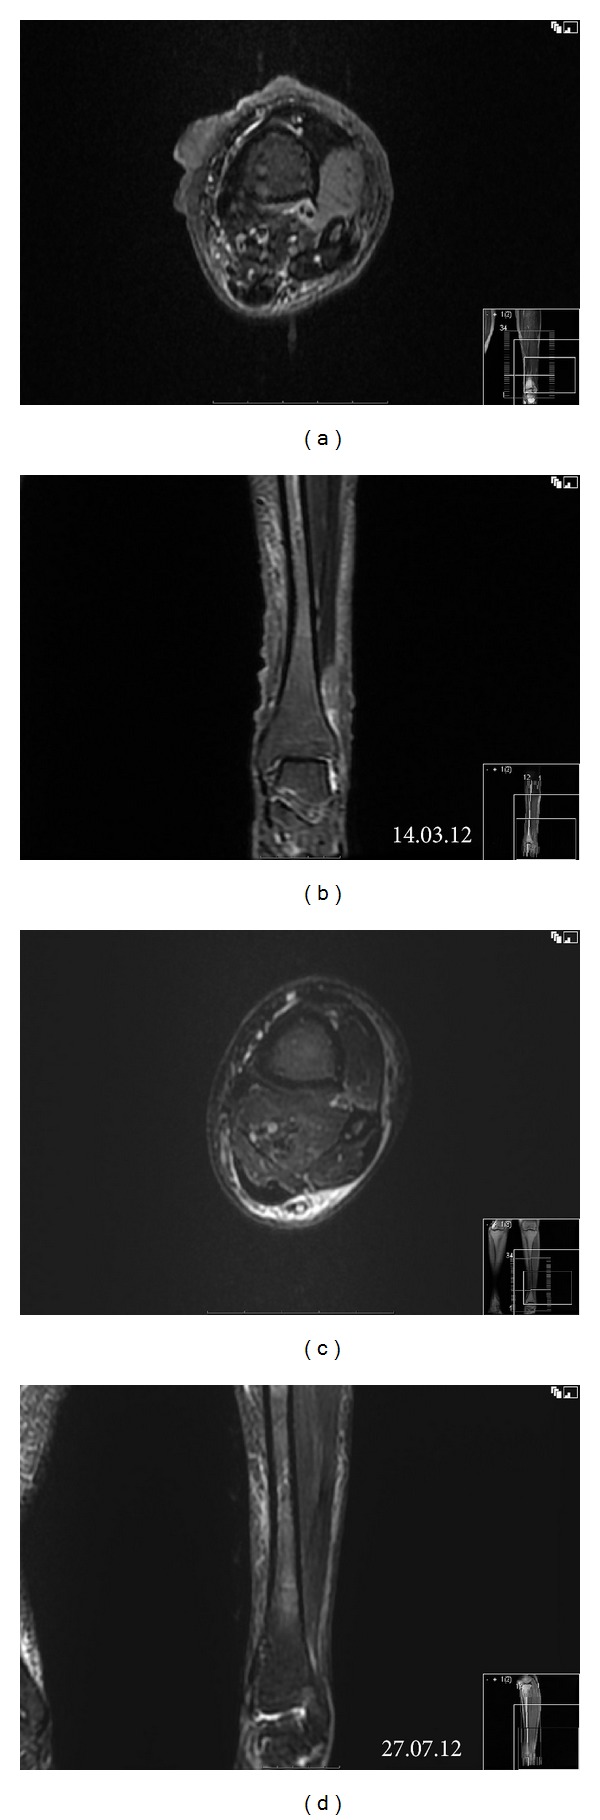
MRI of the left leg. Subcutaneous nodules and myositis of tibialis anterior are shown on T2 fat-saturated axial view (a) and short T1 inversion recovery (STIR) coronal view (b). After treatment, the changes are resolved (c, d).
